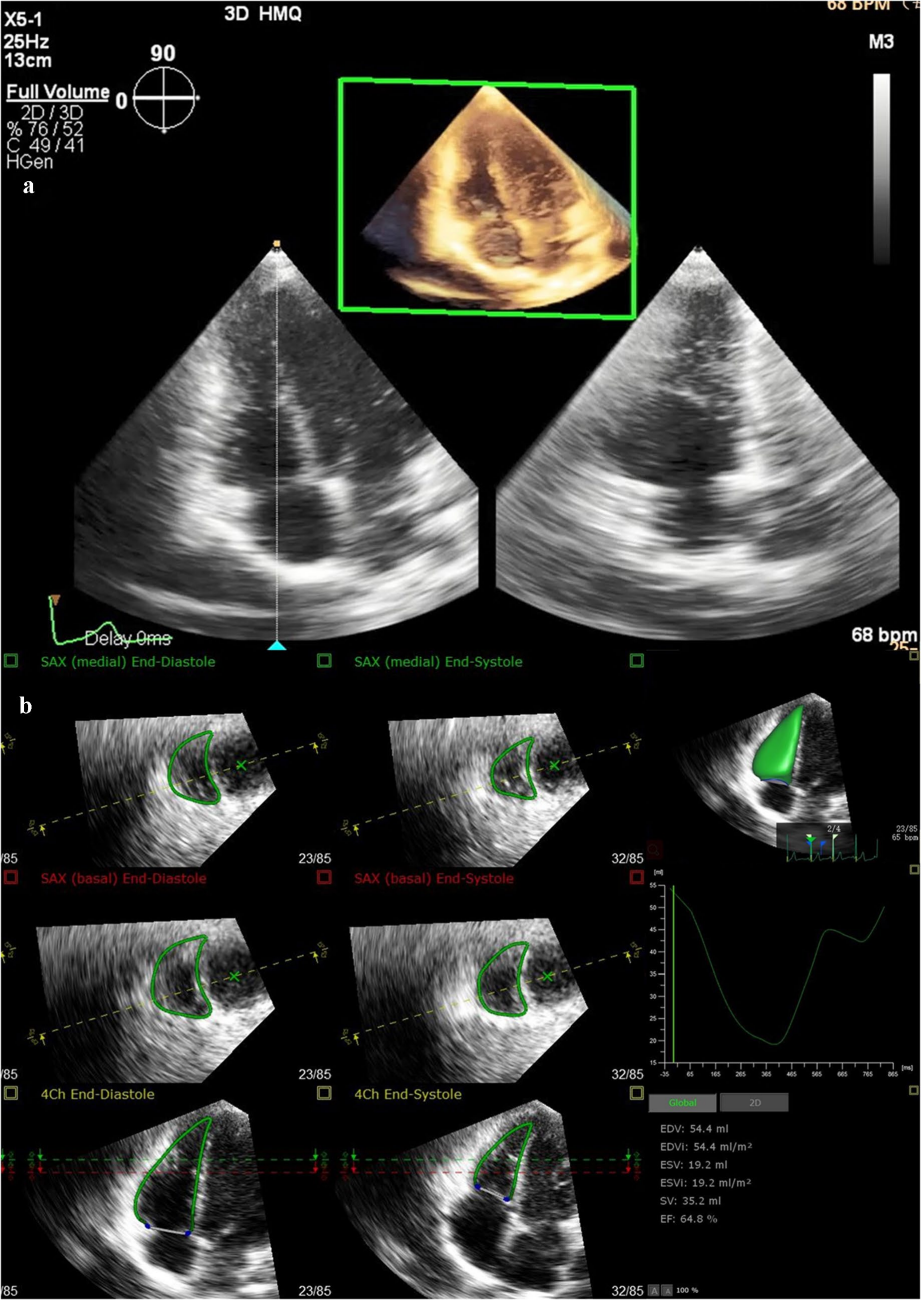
Three-dimensional echocardiography (3DE) depicting the right ventricle in a 6-year-old boy with noncompaction cardiomyopathy. a The 3DE images were obtained on the RV-focused apical four-chamber view in full-volume mode. b Starting the software, the software automatically tracked the right ventricular (RV) endocardial border (*green line*) at the end-diastole and end-systole, and generated a 3DE-RV cast, RV volume curve, and volumetric data
